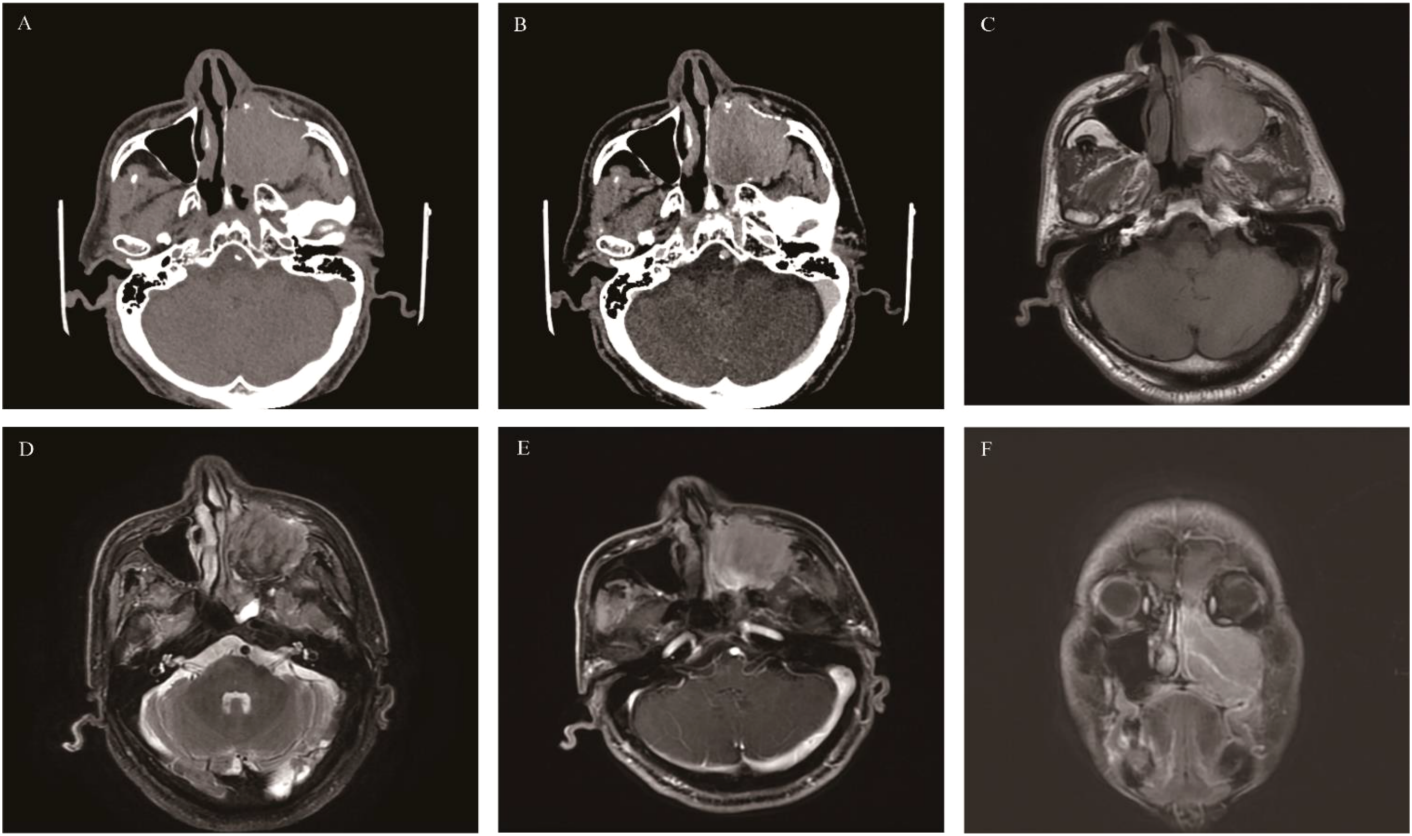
Malignant sinonasal melanoma: An irregular soft-tissue density mass in the left maxillary sinus was accompanied by obvious bone destruction, showing heterogeneous enhancement on CT (A, B); On T1WI, the mass presented isointense signals with linear slightly hyperintense signals within it(C). On T2WI, the slightly hyperintense mass contained multiple linear hypointense areas (D). The mass showed obvious heterogeneous enhancement on axial and coronal MRI (E, F).
